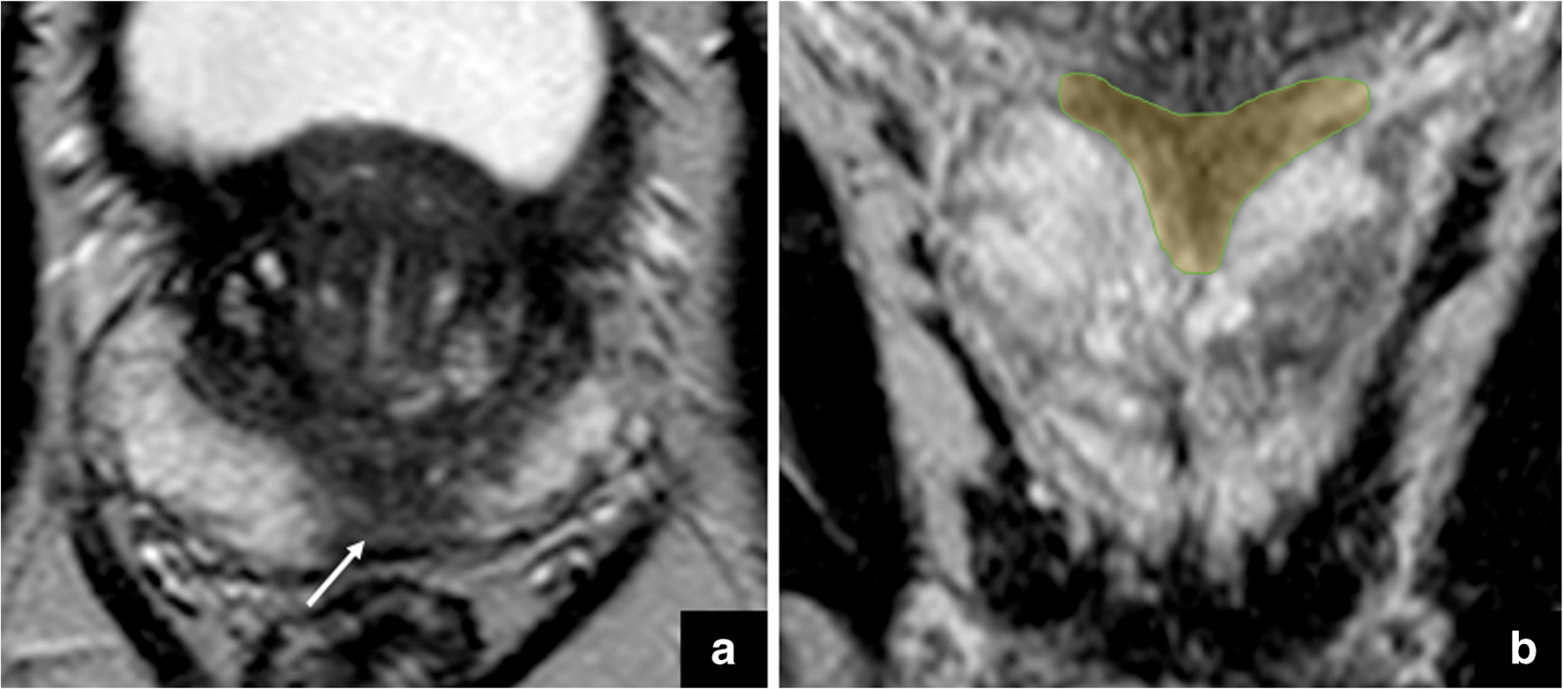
Axial T2-weighted image (a) that shows a hypointense area at the prostate base, in the peripheral zone. This aspect is a variant/extension of the *moustache sign*, in which the central zone is compressed between the transitional and peripheral zones, adopting a *teardrop* shape, as represented by the yellow area in the coronal T2-weighted image (b)
